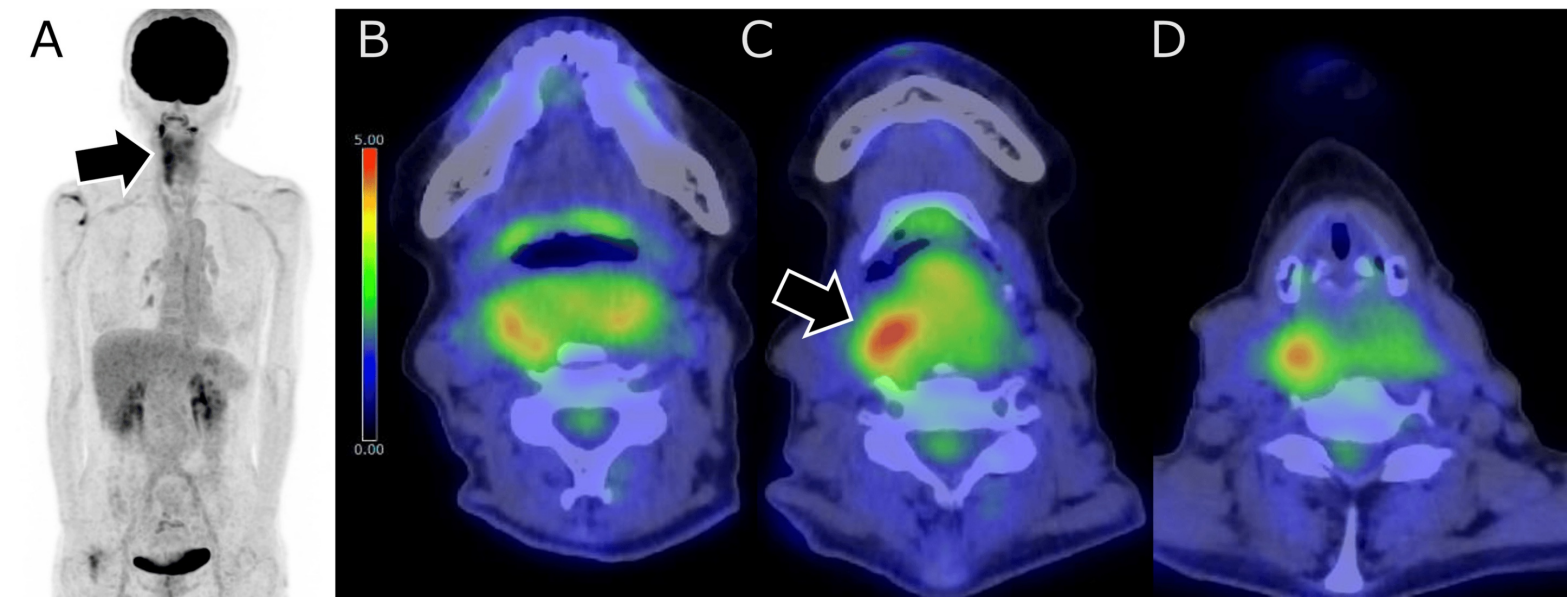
FDG PET/CT findings of the whole body and the lesion. (A) The maximum intensity projection image shows high FDG uptake in the cervical region (black arrow), with no abnormal uptake detected elsewhere in the body.
